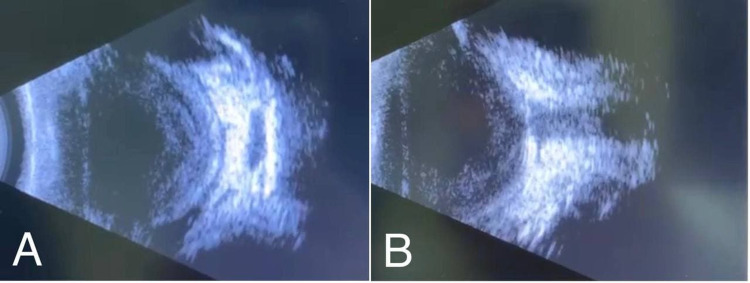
(A, B) B-scan ultrasound pictures of the left eye demonstrate dense infiltrates in the vitreous cavity mostly peripheral, choroid, and retina with subretinal fluid without evidence of shadowing.
